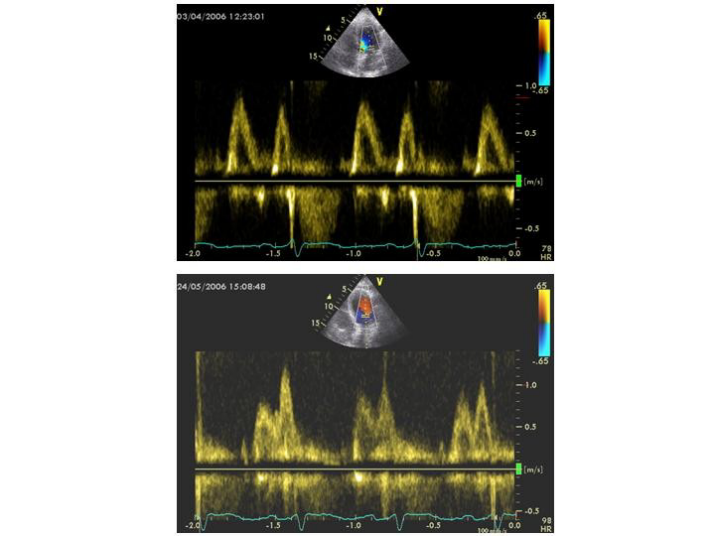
Doppler transmitral inflow pattern in a 58-year old female patient with acute myeloid leukemia before treatment (upper panel) and after DNR (= daunorubicine) 50 mg/m^2^, VP16 (= etoposide) 50 mg/m^2^, ARA-C (= cytarabine) 30 mg/m^2 ^(lower panel). Transmitral pattern, normal before treatment (transmitral E/A ratio = 1.12, deceleration time = 172 ms), shifts to pattern of LV abnormal relaxation, corresponding to grade I of LV diastolic dysfunction (E/A ratio = 0.70, deceleration time = 230 ms) after CT.
